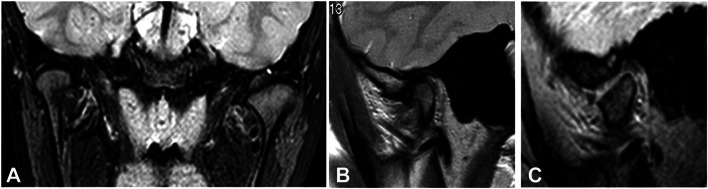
Images of TMJ MRI showing both TMJs in coronal view (A), right TMJ in sagittal view (B) and left TMJ in sagittal view(C)
